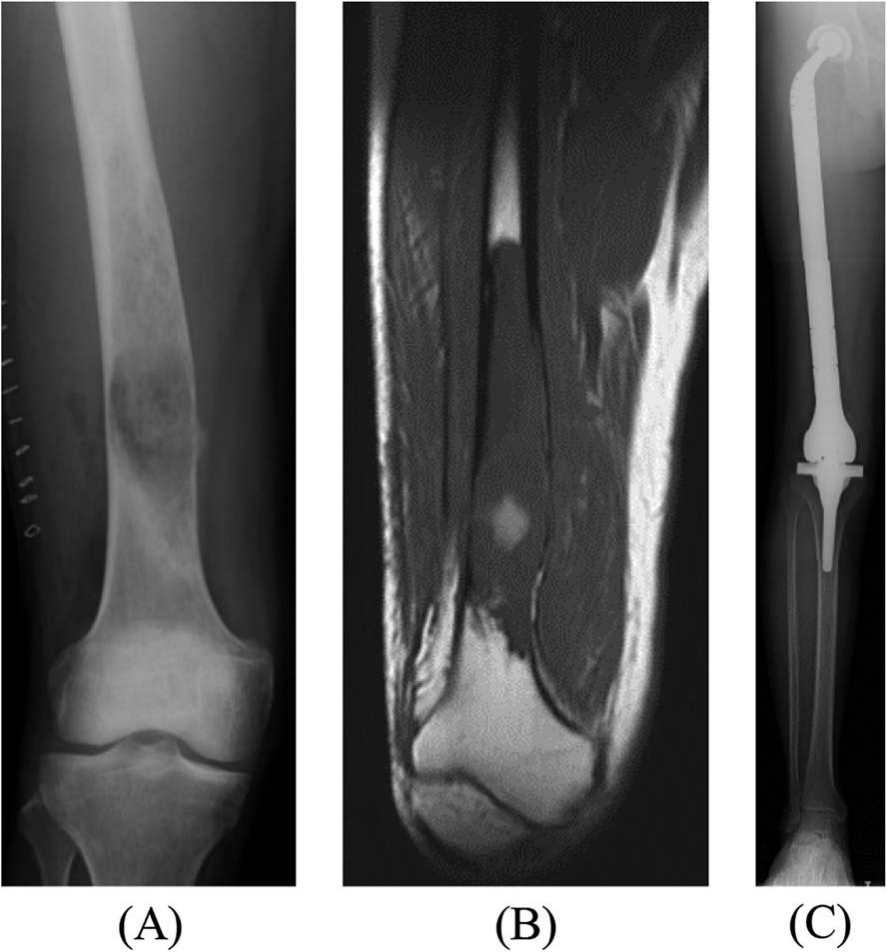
(A) X-ray of the right distal femur of 33-year-old man with a low-grade osteosarcoma. (B) Preoperative T1-weighted MRI showing the involvement of osteosarcoma in the distal two-third of the femur. (C) Radiograph demonstrating reconstruction of total femur after TFR.
